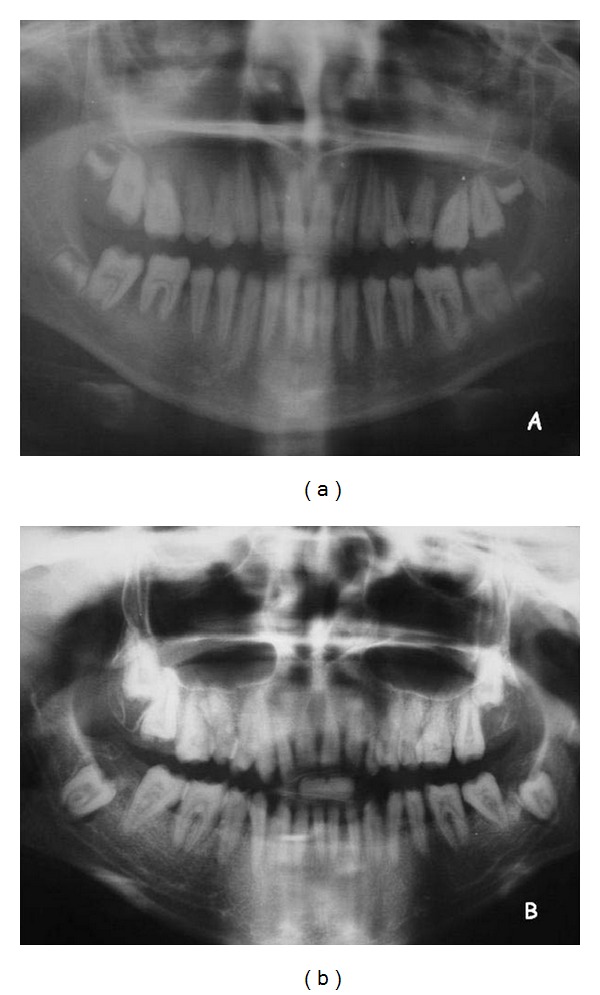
Panoramic radiography shows reduction of enamel thickness for younger (a) and older (b) patients.
